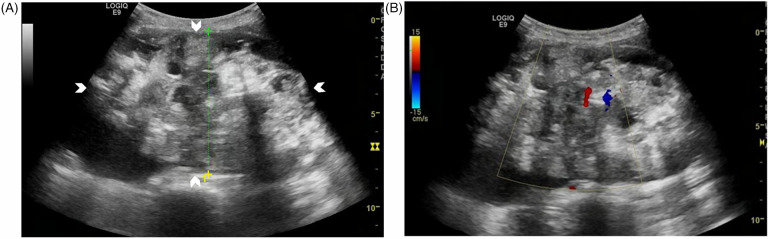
Ultrasound images from a 12-year-old girl with a confirmed immature teratoma. (A) Conventional ultrasound image shows a 13.9 cm irregular outer contour solid lesion (arrowheads). (B) Color Doppler image shows minimal vascularity (Color score = 2). The mass was classified as O-RADS 5.
